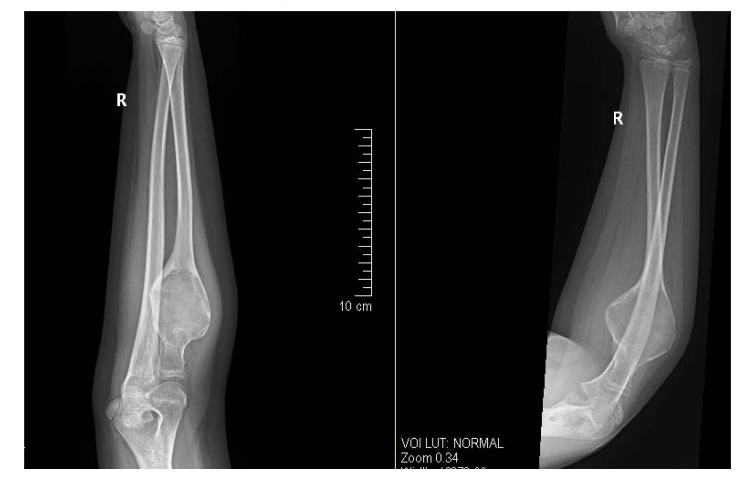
Pre-operative radiograph showing expansile osteolytic lesion of proximal radius, benign in nature with differential diagnosis of aneurysmal bone cyst or simple bone cyst.
